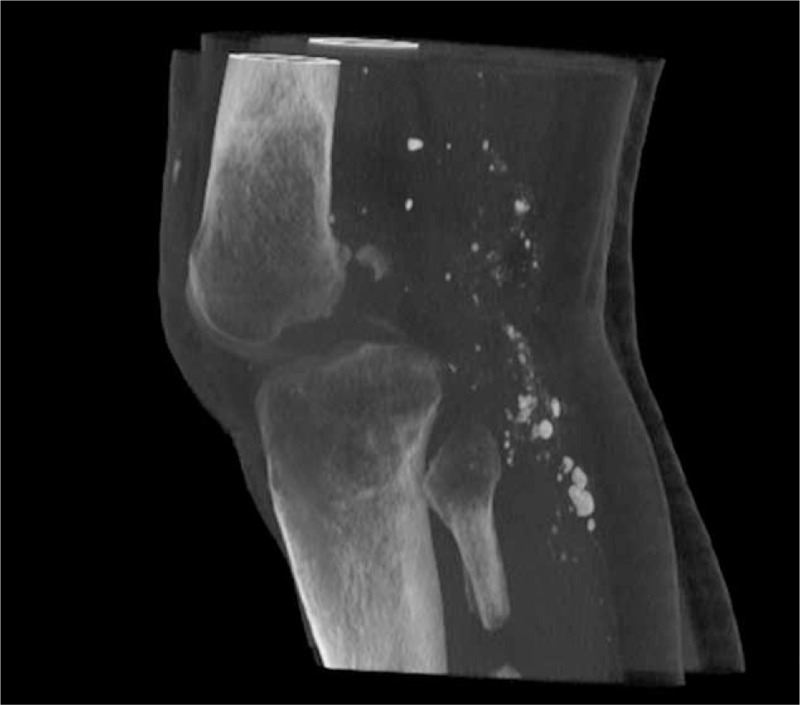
The computed tomography lateral scan of the knee before arthrodesis.
